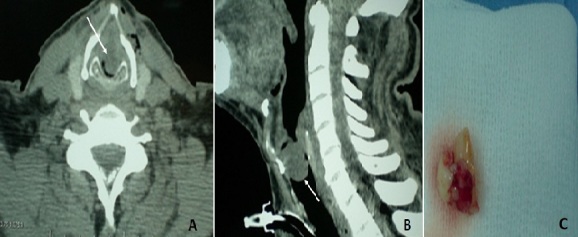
A) axial computed tomography image showing a huge subglottic mass (arrow); B) sagittal computed tomography image showing a homogeneous regular obstructive formation obstructing the glottis and subglottis lumen (arrow); C) excision of the large polypoid mass
